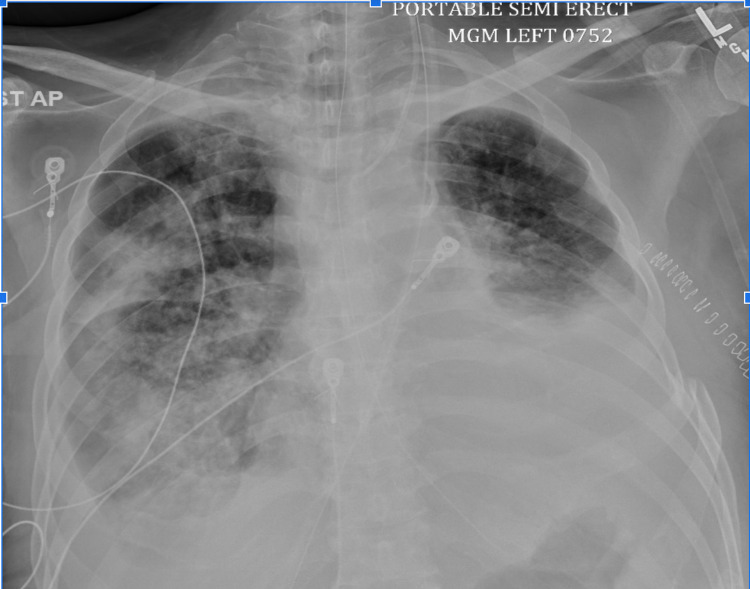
First chest X-ray on admission
